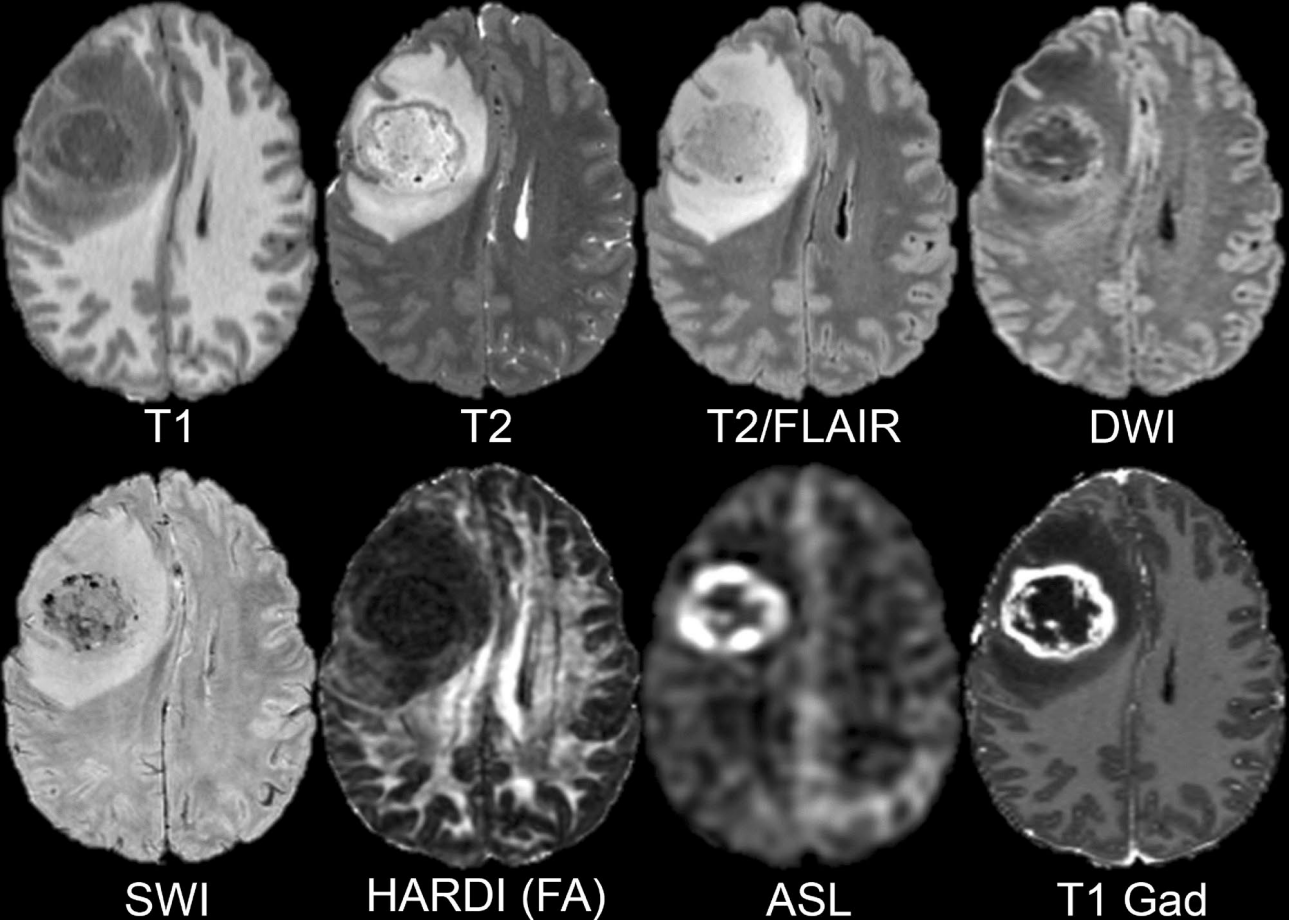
Example axial slices from a pre-processed dataset after non-linear co-registration, resampling, and skull stripping. Eight different image contrasts are shown including T1-weighted pre-contrast (T1), T2-weighted (T2), T2/FLAIR-weighted (T2/FLAIR), diffusion-weighted (DWI), susceptibility-weighted (SWI), HARDI fractional anisotropy (HARDI FA), arterial spin labeling perfusion (ASL), and T1-weighted postcontrast (T1 Gad).
